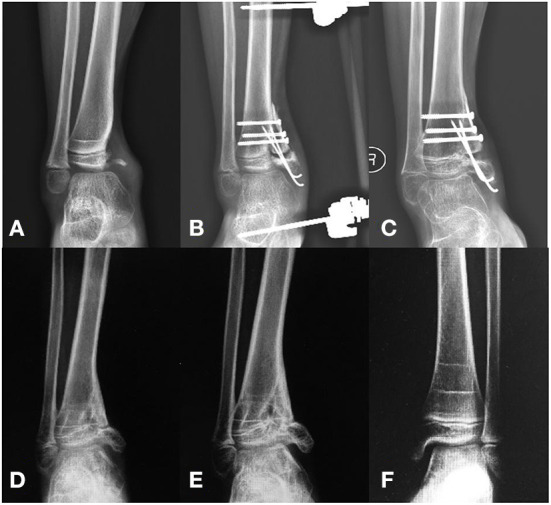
Anteroposterior X-ray view of a 7.5 years old boy shows the ankle joint in varus at 20 months after the Salter type VI physeal injury (A). Post-operative X-ray shows a wedge-shaped iliac apophyseal bone autograft (B). Five months post-operative X-ray shows the united iliac crest apophyseal autograft (C). A physeal bar on the distal medial tibia was observed 20 months after reconstructive surgery (D). On 36 months follow up after reconstructive surgery, the reconstructed medial malleolus (E) compared with the contralateral side (F).
